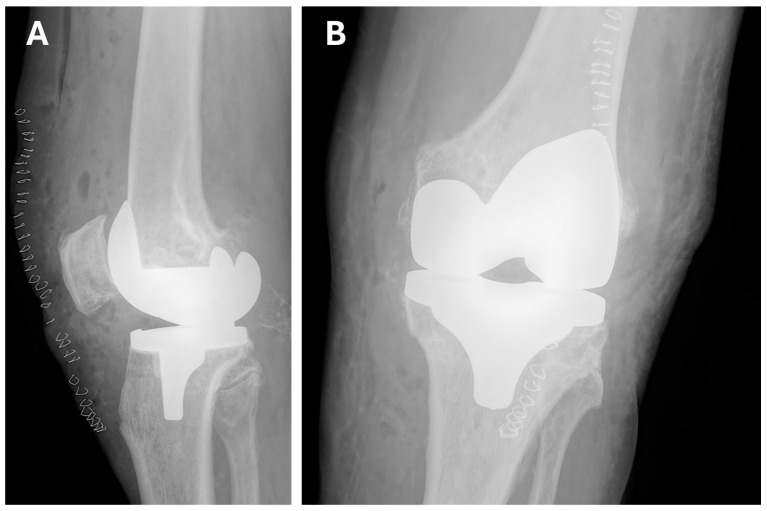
Postoperative X-ray of the left knee joint—day 1 after TKA. (A) Lateral plane. (B) Anteroposterior (AP) plane. The correct positioning of the femoral and tibial components is visible. The mechanical axis of the joint has been restored.
